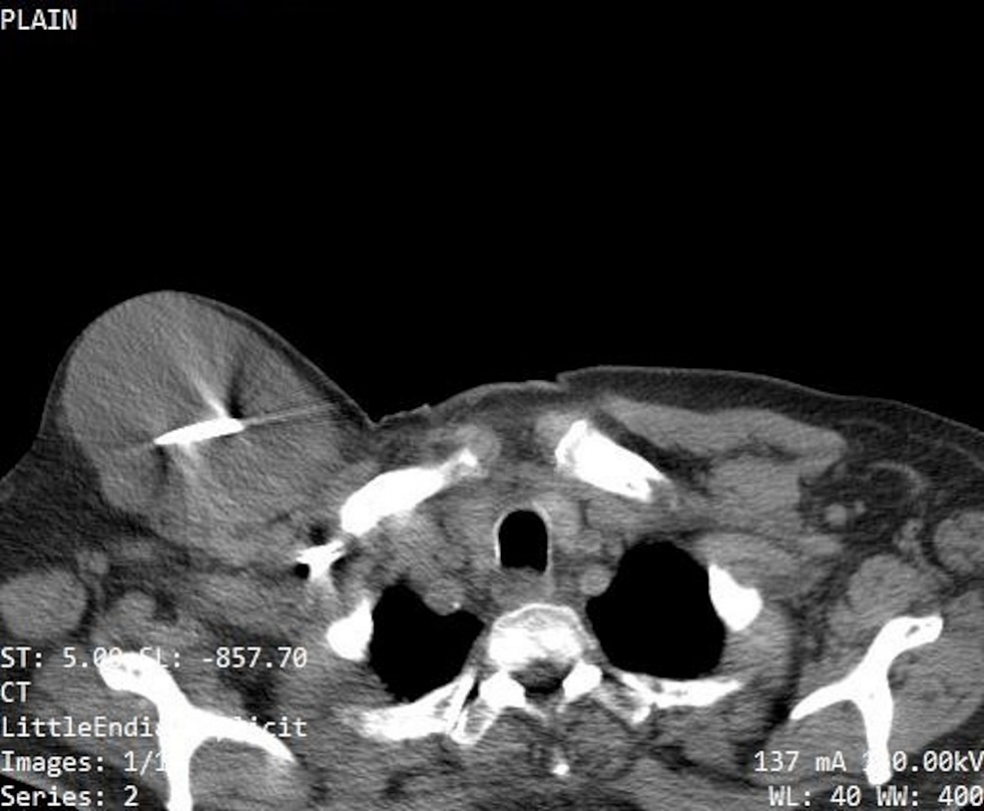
CT scan showing collection/seroma measuring 6.7 x 9.4 x 11 cm in the pacemaker insertion site. Pacemaker generator and lead wires seen within it.
